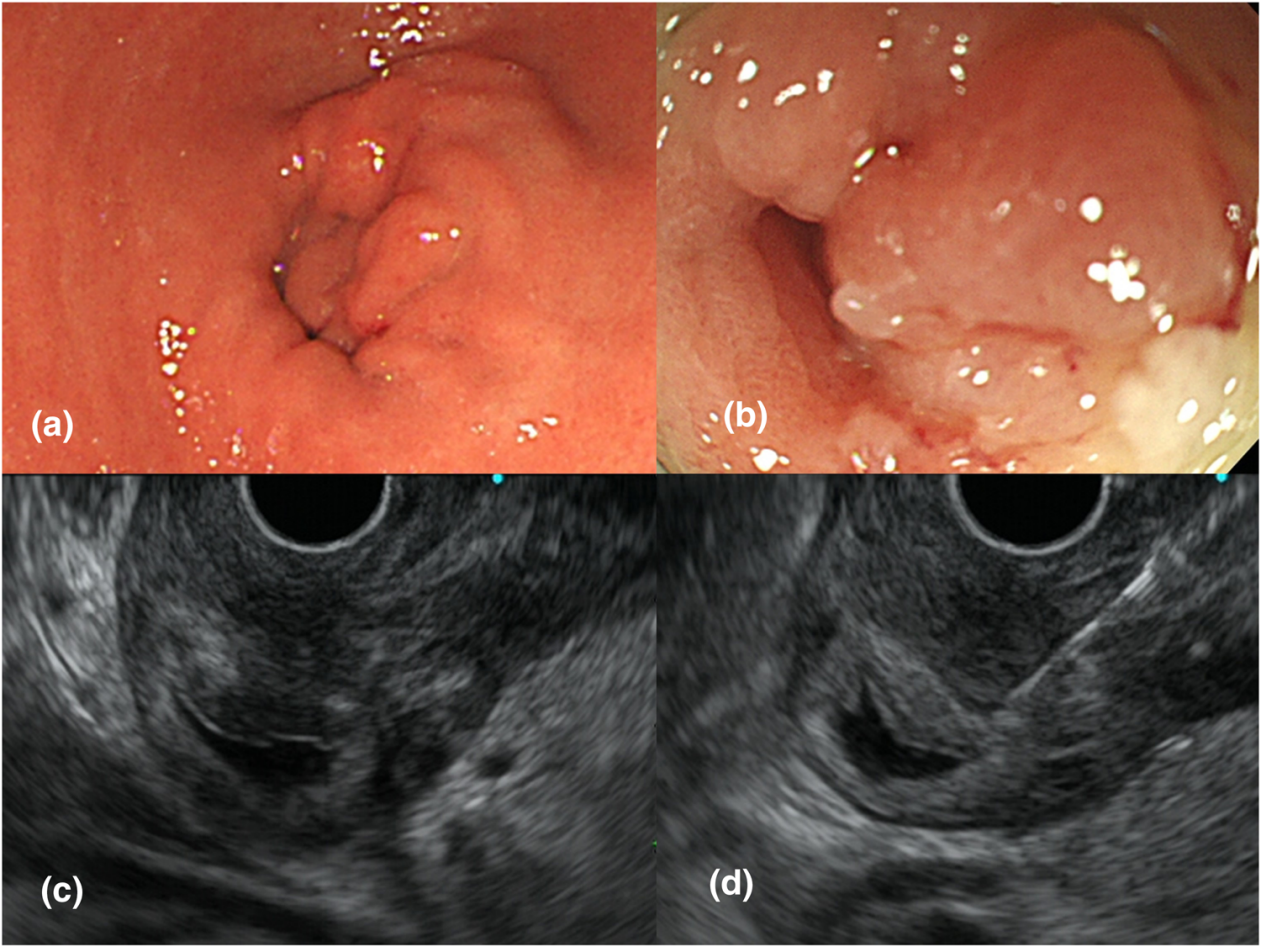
Endoscopic findings: (a, b) esophagogastroduodenoscopy showing mucosal swelling of the antrum and a stenotic site at the pylorus; (c) endoscopic ultrasonography findings showing gastric wall thickening at the antrum; (d) endoscopic ultrasound‐guided fine‐needle biopsy
